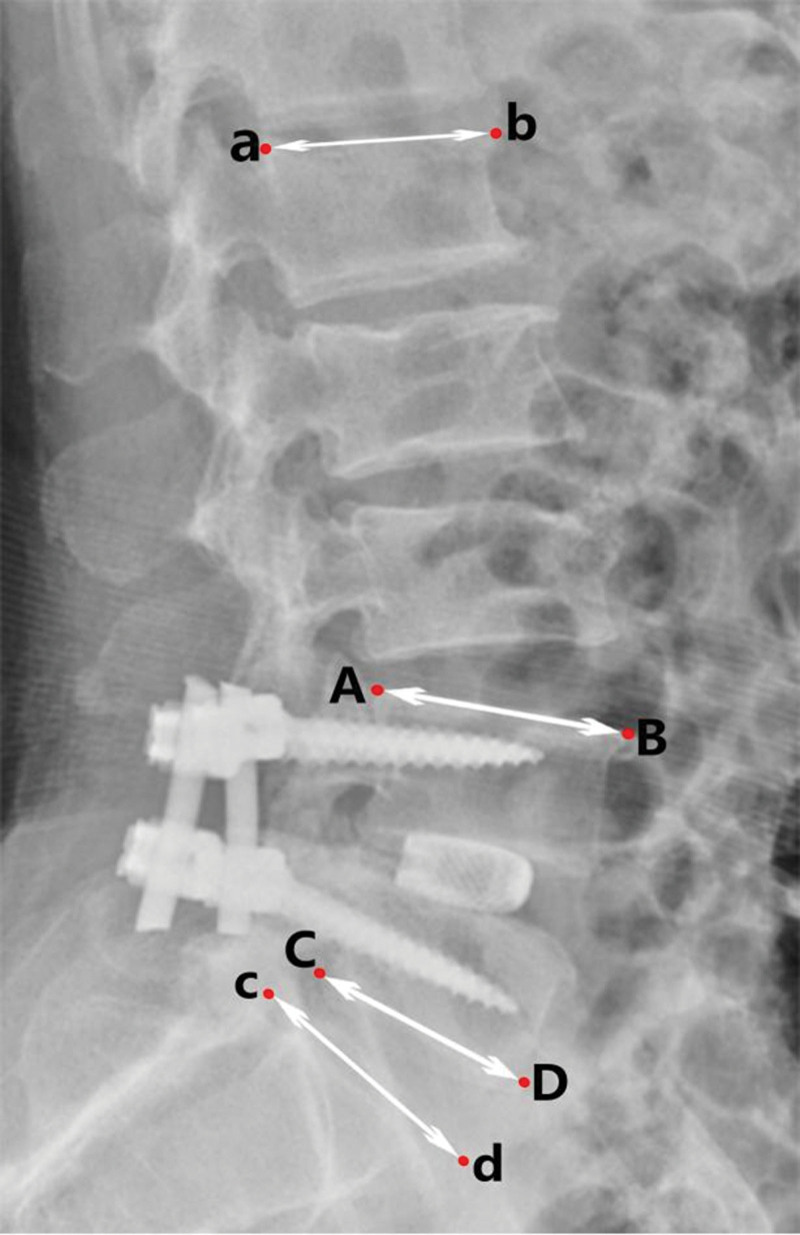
Lateral X-ray of lumbar vertebra. Segmental lumbar lordosis angle: the angle formed by the extension line of segment AB and segment CD. Lumbar lordosis angle: the angle formed by the extension line of segment ab and segment cd.
